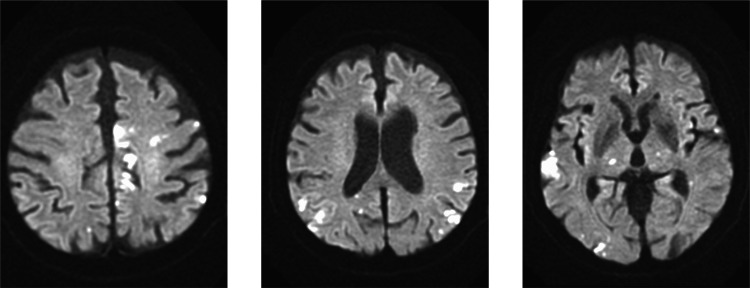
Findings of magnetic resonance imaging (MRI) in case 1 MRI scan reveals multiple cerebral infarctions (diffusion-weighted MRI scan), which developed four days after the second nivolumab administration.
